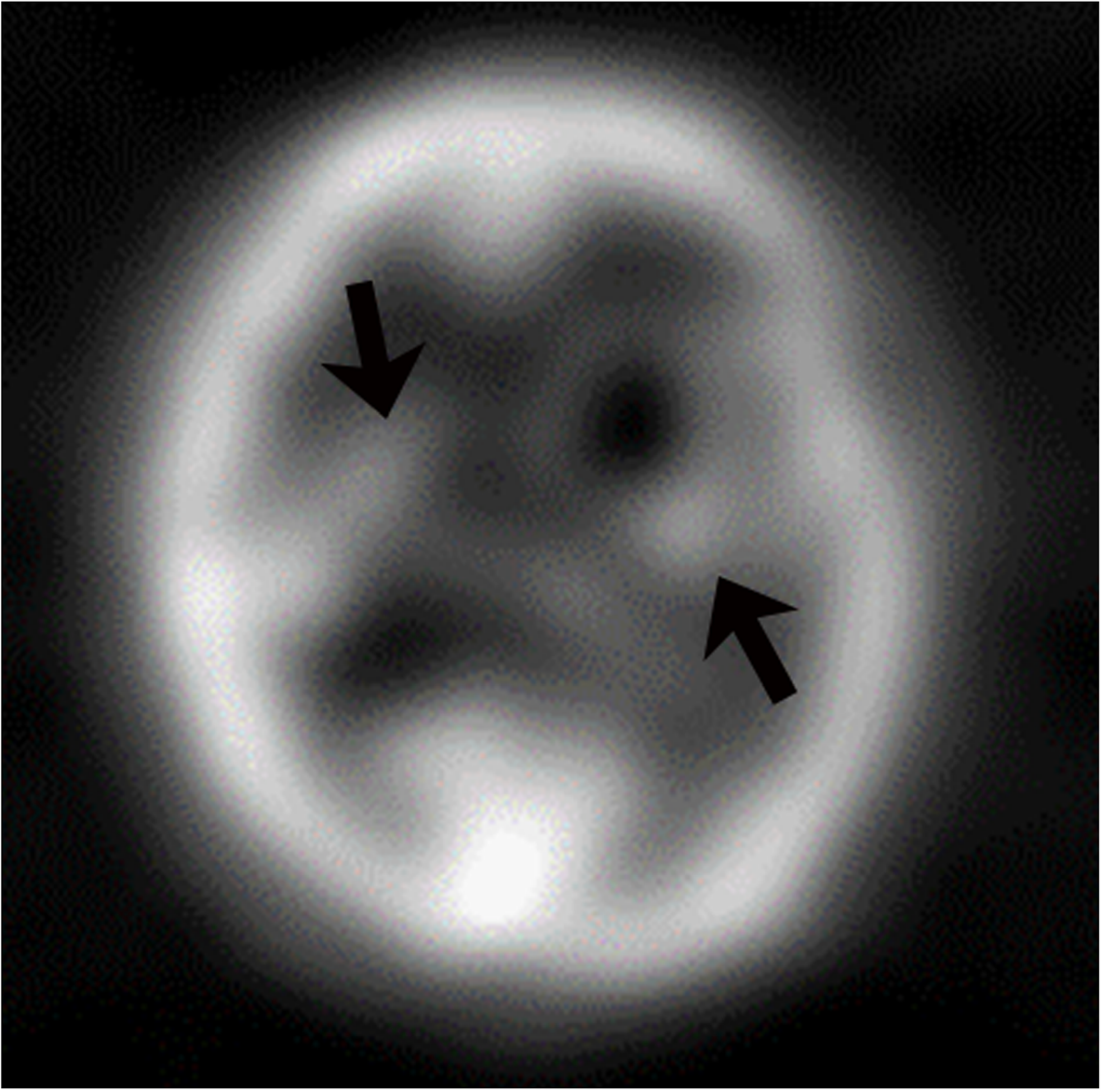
Primary central nervous system post-transplant lymphoproliferative disorder. F-18 FDG PET exam demonstrates bilateral cerebral hypermetabolic lesions in the basal ganglia (arrows). The lesion on the left is larger but the lesion in the right basal ganglia had a higher SUVmax of 8.5 versus SUVmax of 7.2 in the left basal ganglia. Notice how hypermetabolic the cortex is and how this could obscure more peripheral lesions
